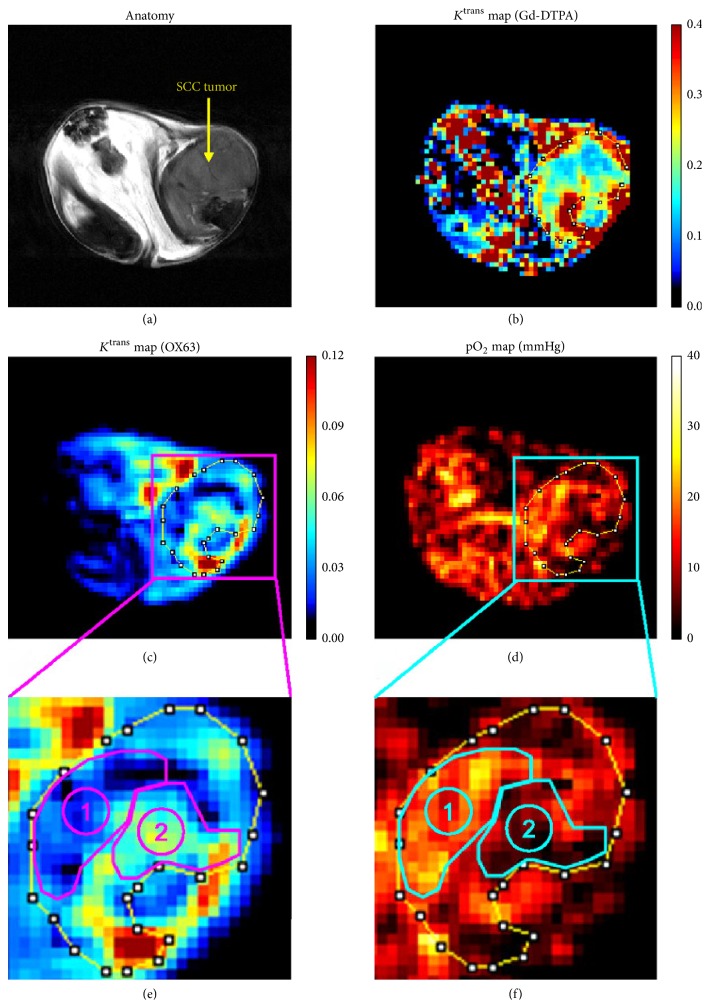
Tumor oxygenation and microvascular permeability using Overhauser-enhanced MRI (OMRI). Comparison of *K*^trans^ maps of Gd-DTPA and OX63 (radical) in a squamous cell carcinoma (SCC). (a) SCC tumor region can be detected in a *T*_2_-weighted image by using 7-T MRI. (b) *K*^trans^ map of Gd-DTPA. (c) *K*^trans^ map of OX63 using OMRI of the same SCC tumor. Note OMRI/OX63 images were obtained before the 7-T MRI/Gd-DTPA study. (d) Corresponding pO_2_ map computed from the same OMRI images for *K*^trans  OX63^ map. ((e), (f)) Based on the anatomical image, ROI of SCC tumor was selected and enlarged. Tumor region with low *K*^trans  OX63^ values (ROI 1) was relatively oxygenated and normal muscle tissue, and the region with high *K*^trans  OX63^ values (ROI 2) coincided with hypoxia in pO_2_ image (adapted from [16]).
